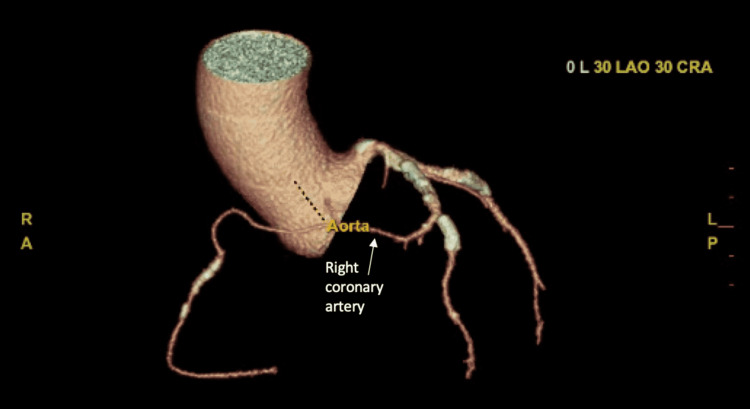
Three-dimensional coronary computed tomography angiography showed the anomalous right coronary artery.
